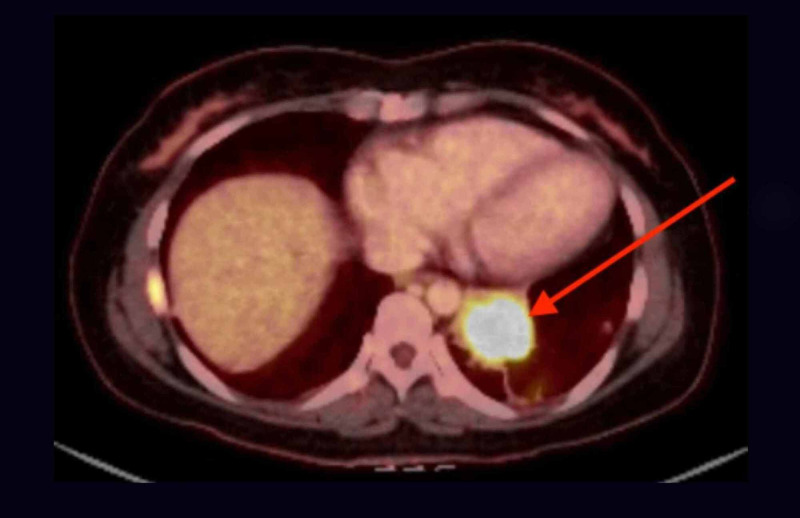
PET/CT showing fluorodeoxyglucose (FDG) avid 4.5 x 4.2 x 3.6 cm LLL mass (red arrow) with max standardized uptake value (SUV) of 12 and FDG avid intramedullary metastasis in the lateral right 7th rib PET/CT: positive emission tomography/computed tomography; FDG: fluorodeoxyglucose; LLL: left lower lung; SUV: standardized uptake value; cm: centimeter
